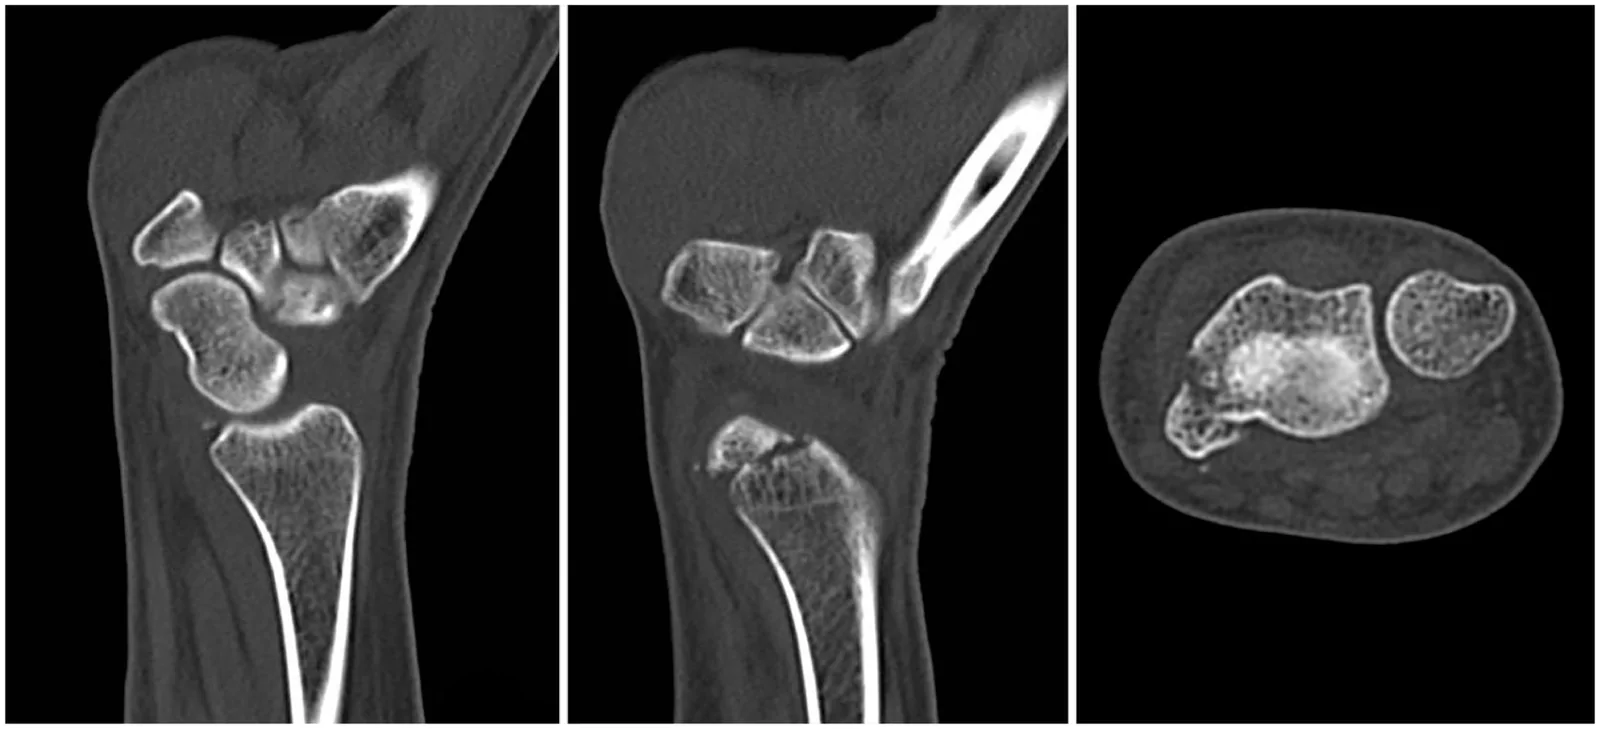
CT showing the small avulsed radial styloid/rim fragment with displacement and associated radiocarpal rotatory subluxation.
